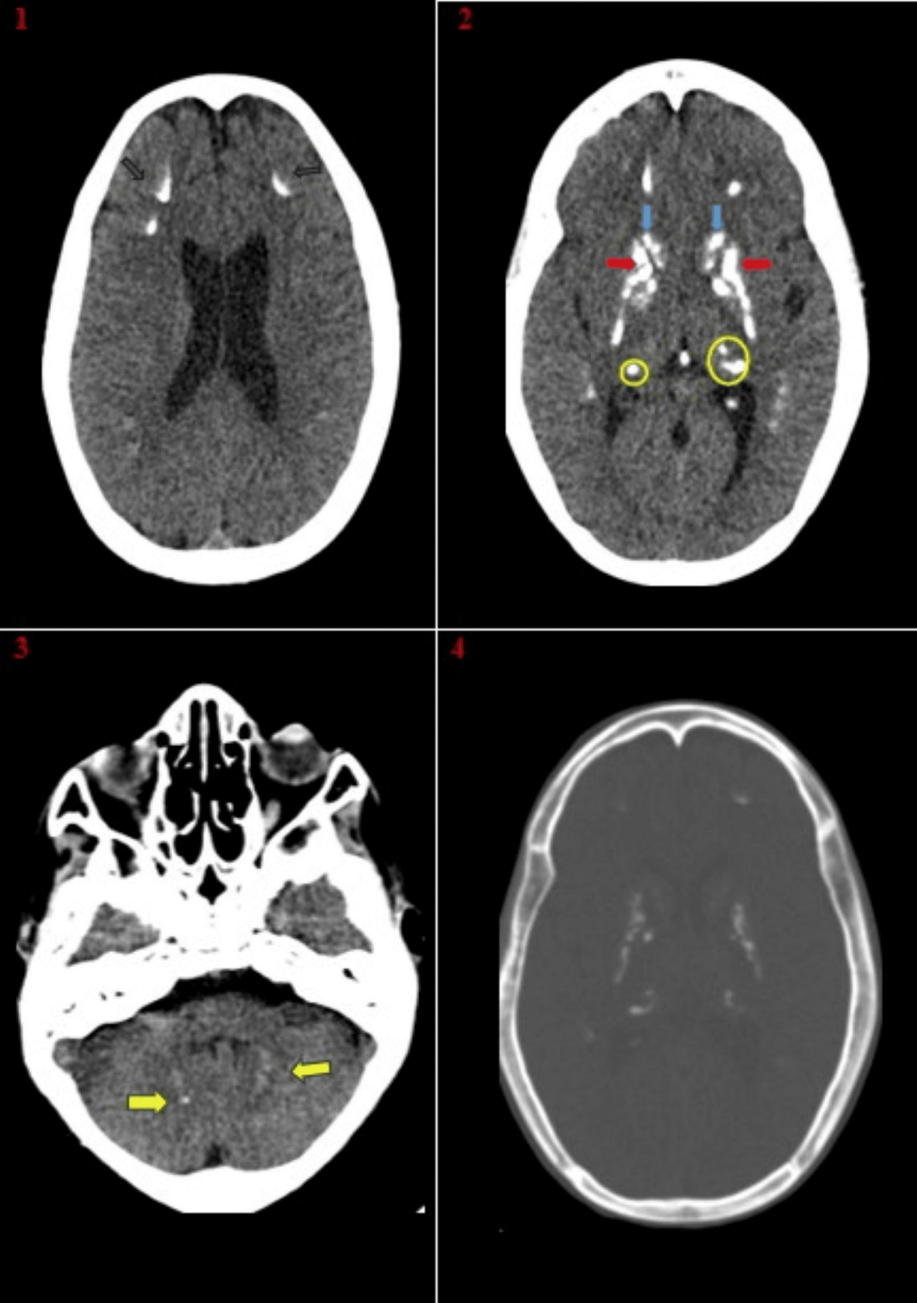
Non-contrast CT of the brain (axial CT images) (1): Arc-shaped calcifications at the junction of gray matter and white matter (arrow). (2): Coarse calcifications localized within the caudate nuclei (indicated by the blue arrow), lentiform nuclei (marked by the red arrow), and pulvinar regions (circled). (3): Fine, low-density calcifications localized within the dentate nuclei of the cerebellum (indicated by the arrow). (4): Non-contrast CT scan using a bone window reveals densities comparable to those of the previously mentioned calcifications.
